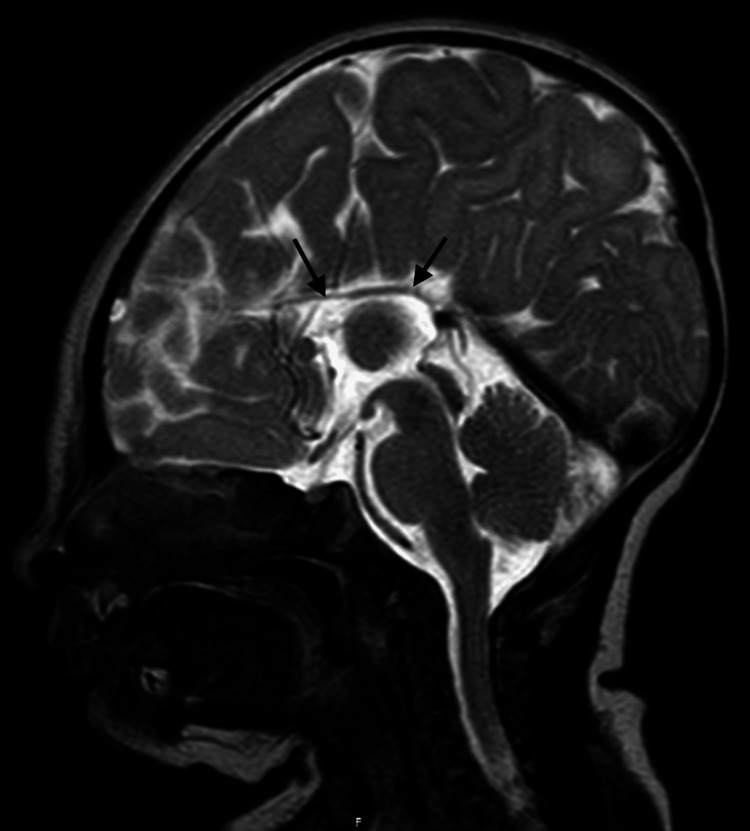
MRI of the brain, T2 sequence in the sagittal plane, demonstrating complete agenesis of the corpus callosum. The remaining brain parenchyma appears normal, with mild thinning of the white matter observed. Source: WellSpan York Hospital.
